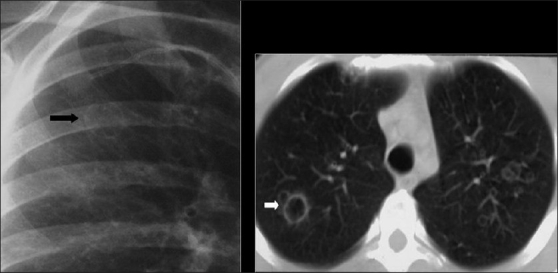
Cryptococcosis. Chest X-ray (L) shows a thin-walled cavity (arrow) associated with patchy consolidation. Computed tomography section at the level of the lower trachea (R) shows multiple cavities of varying sizes associated with subtle ground-glass opacification
